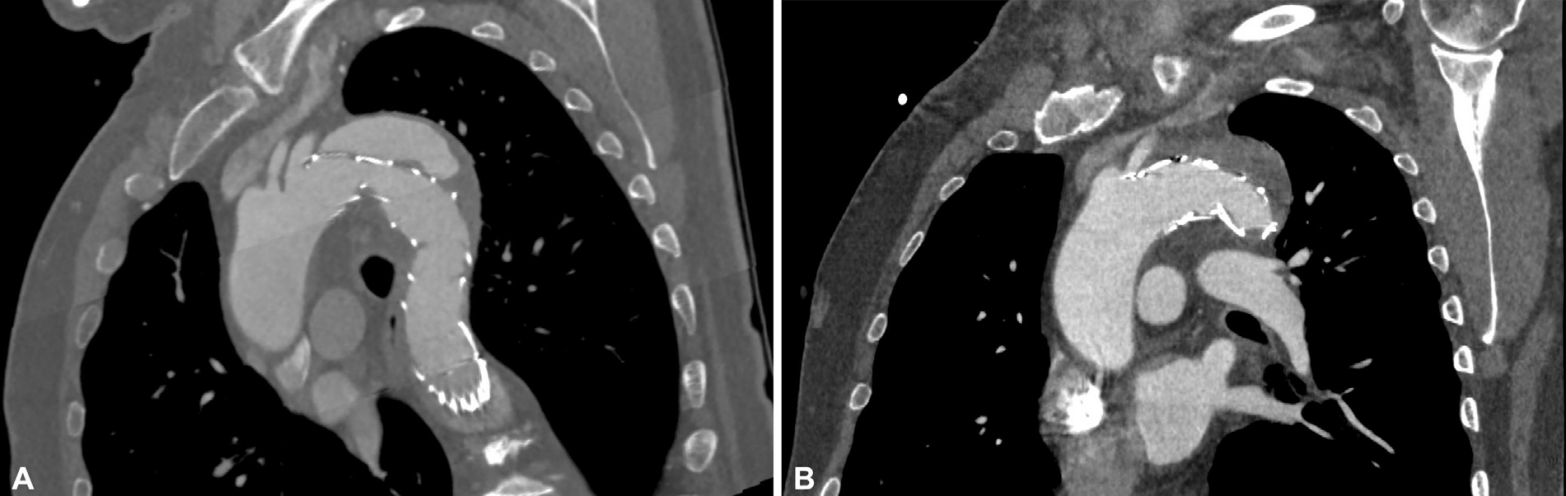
Representative computed tomography angiography of a type IA endoleak (
